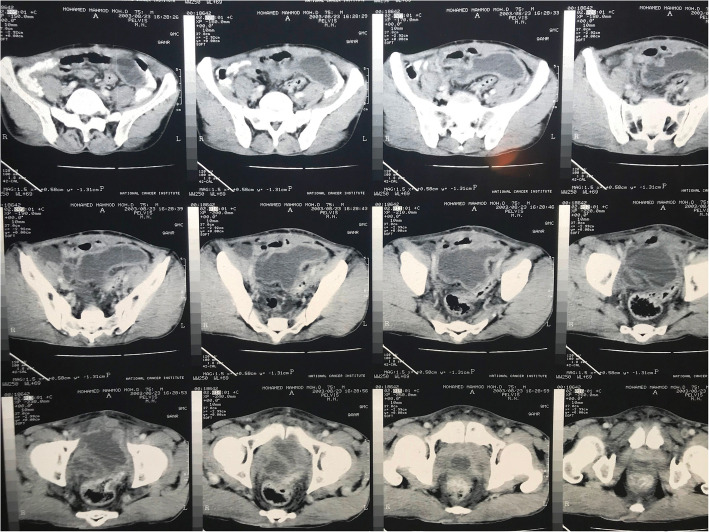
Post-evacuation CT cuts, 23 months post-RC and W diversion. There is pouch over distension and large 250 ml residual
